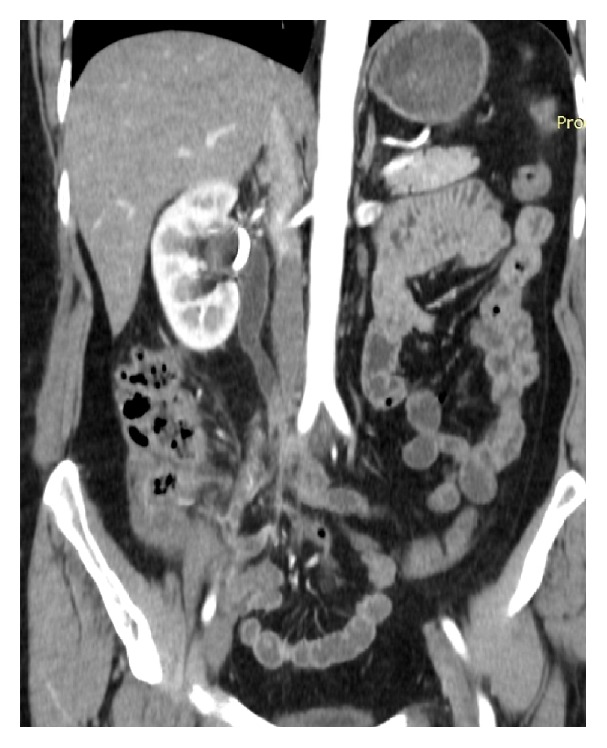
Hydronephrosis on abdominal CT, coronal plane.
